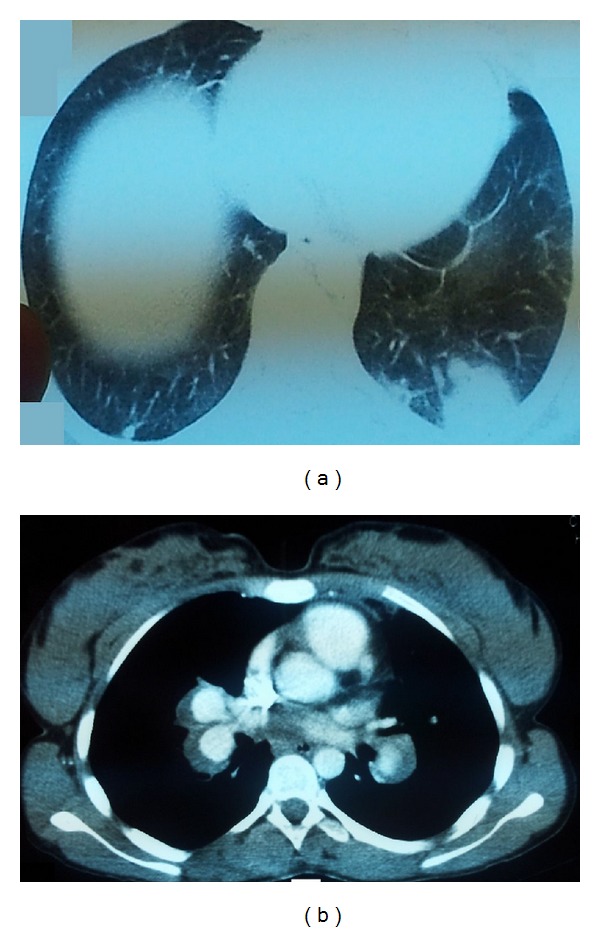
Thorax computed tomogram showing consolidated substances related to emboli in the posterobasal segment of the left pulmonary lower lobe (a), pulmonary artery thrombus and aneurysmal dilatation of the pulmonary artery (b).
